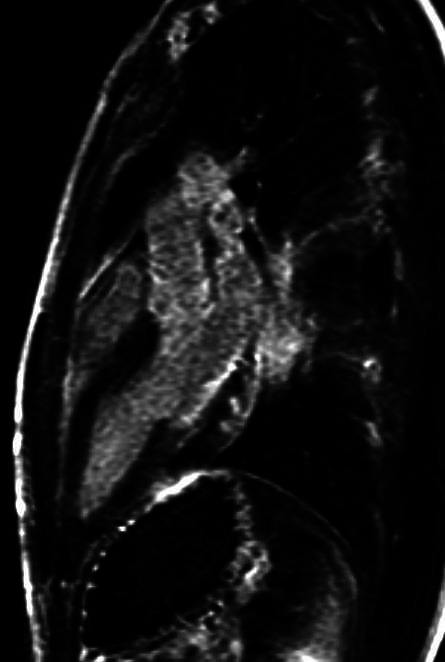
MRI image analysis. Postcontrast images: late images, evidence of enhancement areas with subepicardial/intramyocardial distribution in the basal segments of the inferior wall.
